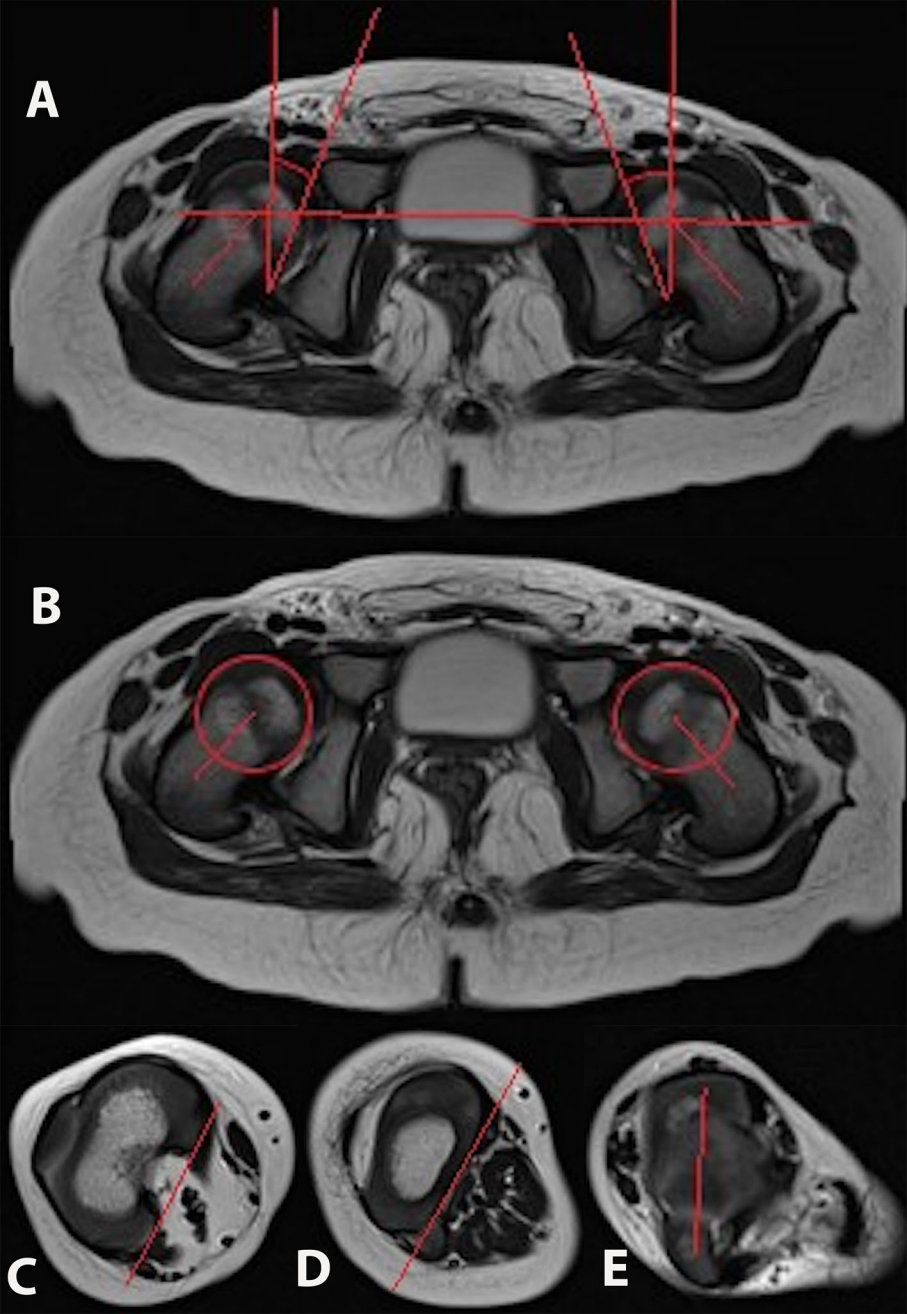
Axial/frontal MRI sections with measurement and reference lines of acetabular anteversion (A), acetabular coverage (A), proximal femur (B), distal femur (C), proximal tibia (D), and bimalleolar axis (E).
